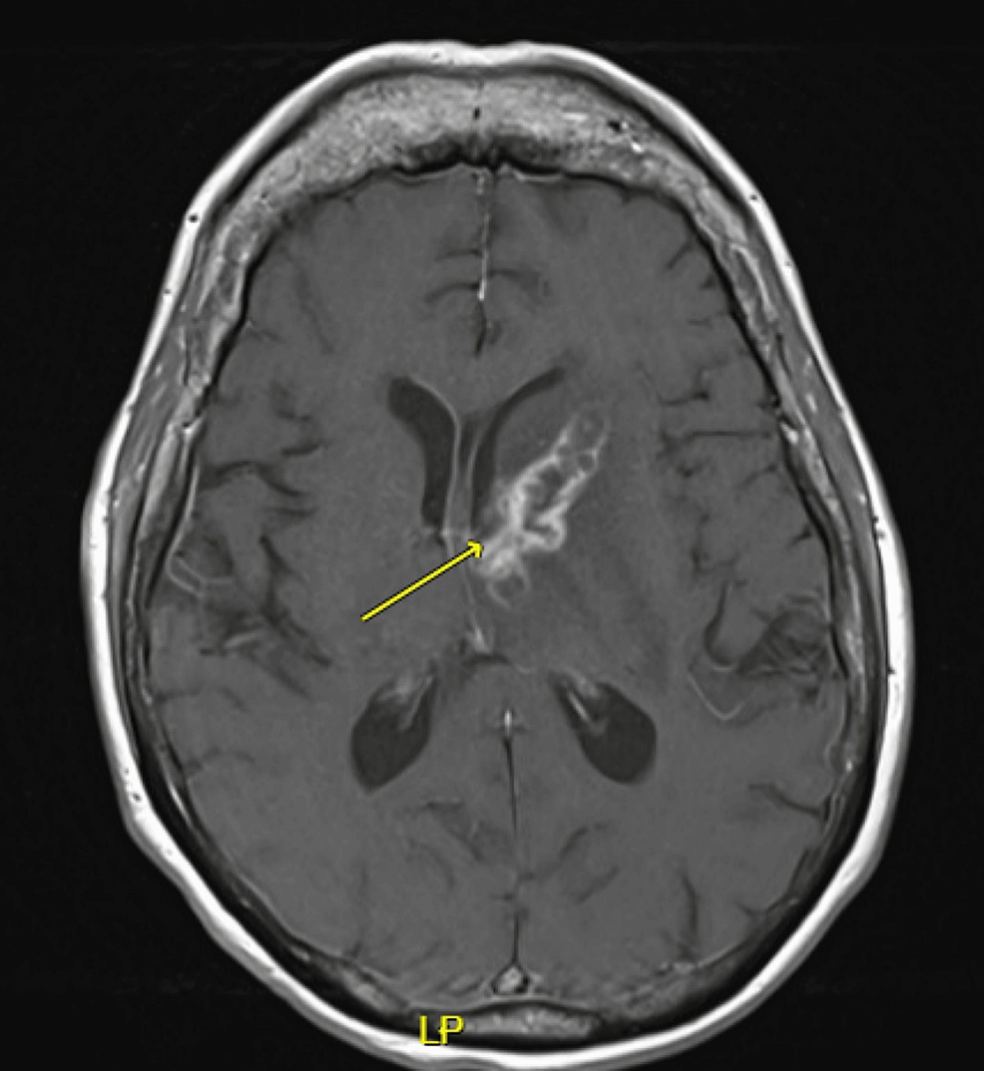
An axial T1-weighted MRI brain image displays a lesion with peripheral enhancement located in the left basal ganglia. Additionally, a smaller, similar lesion in the right temporal lobe is not visible in this image. Mild to moderate vasogenic edema was observed surrounding both lesions on T2-weighted imaging MRI: magnetic resonance imaging
